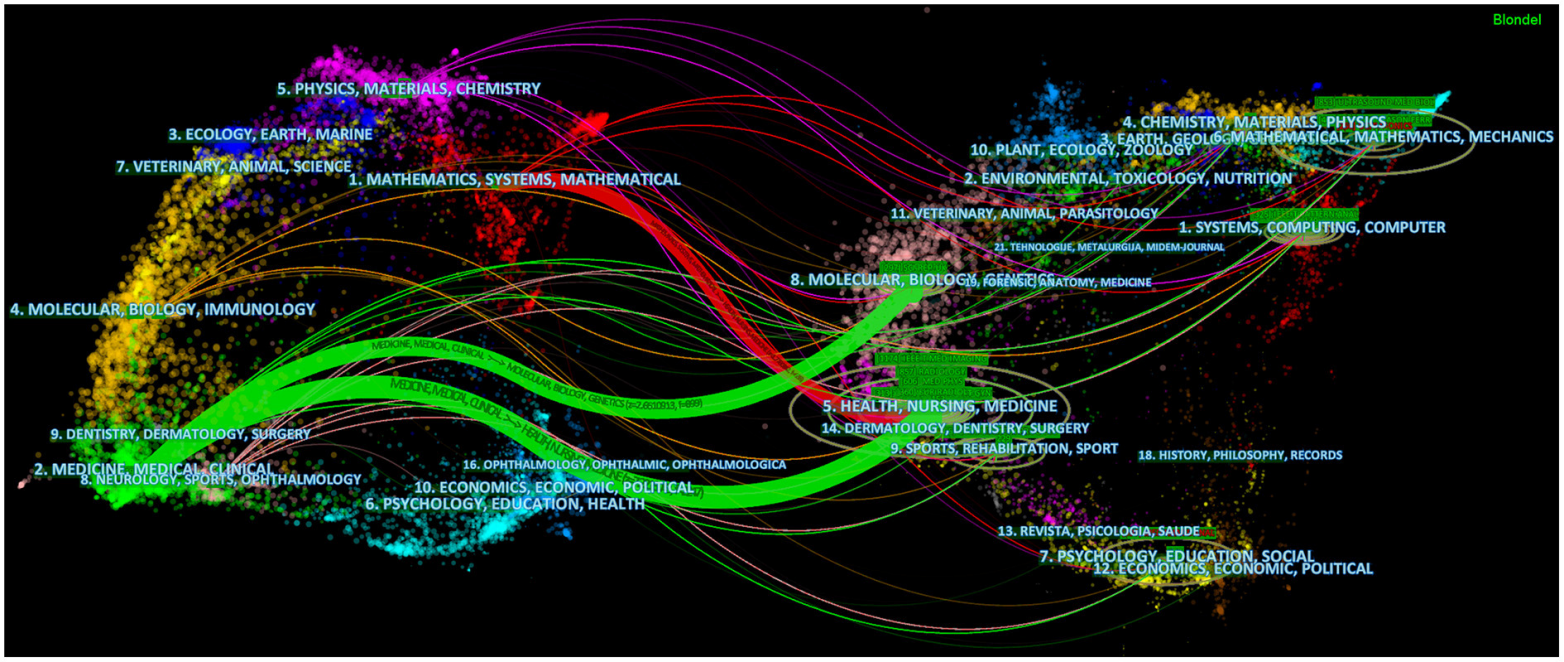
Dual-mapping overlay about AI applied in the field of ultrasound. The left side represents the fields of articles included in the study, and the right side represents the fields of references of articles.
